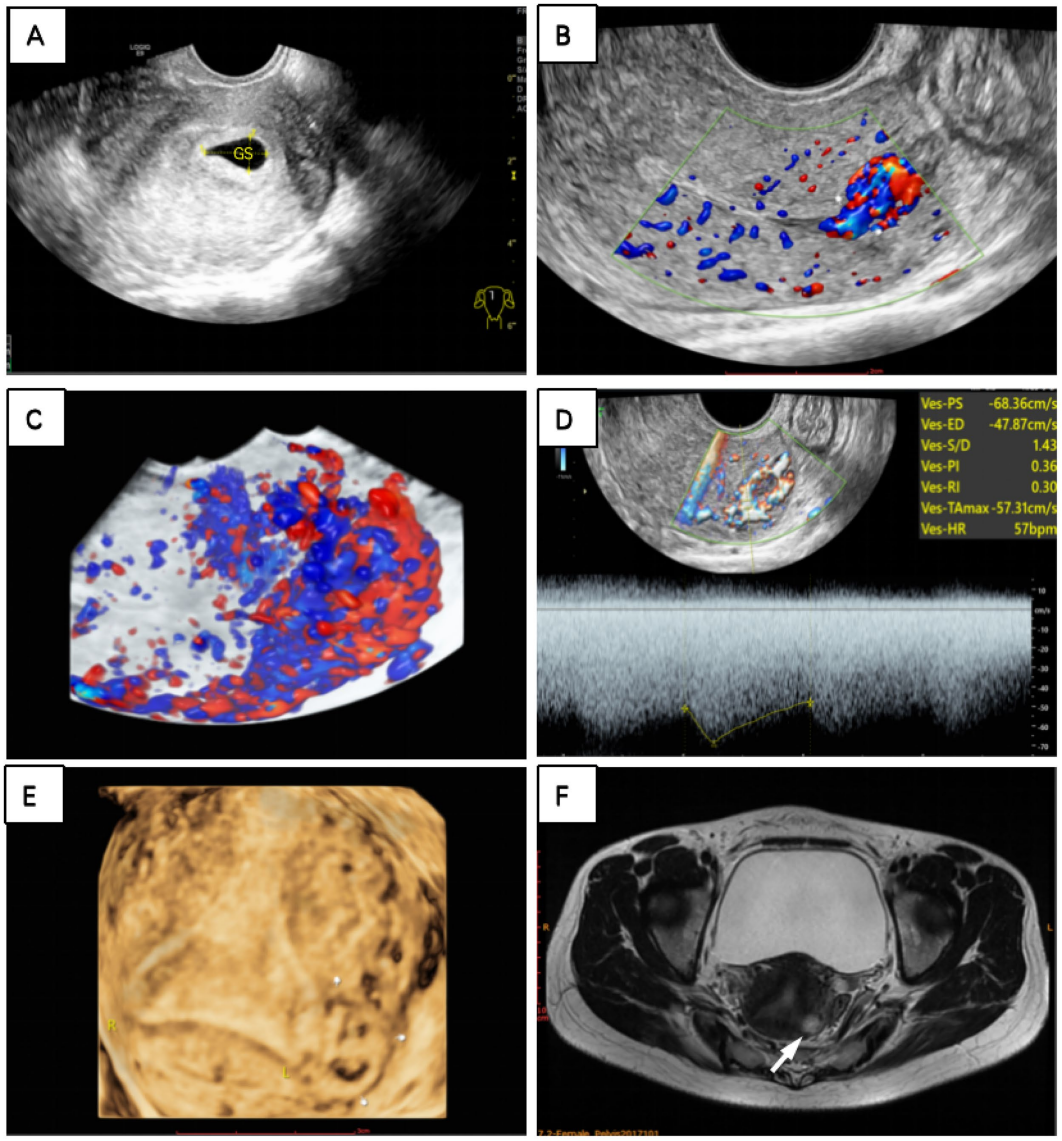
(A) A hypoechoic area was observed in the uterus on transvaginal ultrasound. Two months after medication abortion, (B) CDFI and (C) three-dimensional flow imaging revealed abundant blood flow signals resembling vascular pools at the uterine fundus. (D) Spectral Doppler revealed high-speed, low-resistance arteriovenous fistula-like flow. (E) Three-dimensional ultrasonography and (F) MRI T2-weighted sequence revealed a gestational sac within the left myometrium, outside the endometrial cavity (white finger and arrow pointing to the gestational sac).
